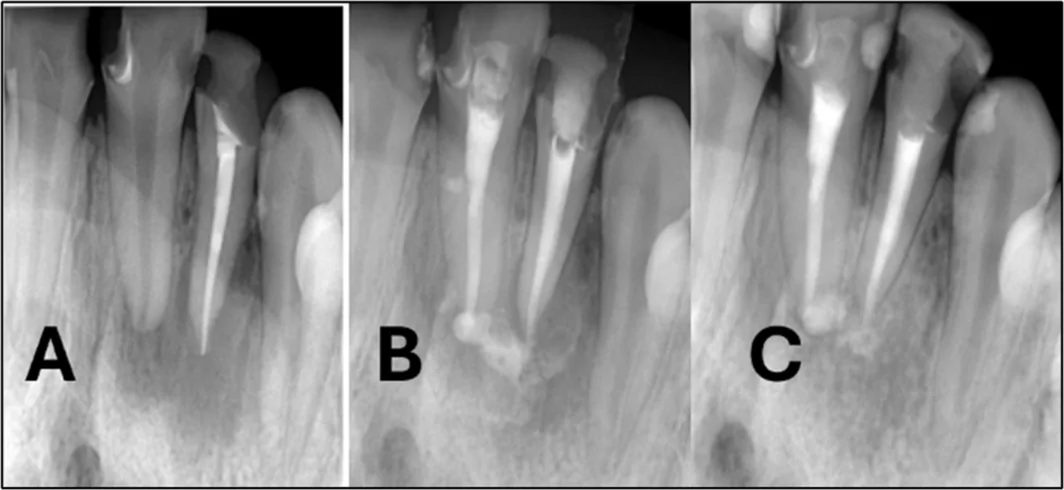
Case 2. (A) Preoperative periapical radiograph demonstrating a large periapical radiolucency involving teeth #23 and #24. (B) Immediate postoperative radiograph following placement of 5‐mm CC apical plugs, with extrusion of CC. (C) Two‐year follow‐up periapical radiograph showing that the previously observed periapical radiolucency was no longer evident and that the radiographic visibility of the extruded CC was reduced compared with the immediate postoperative image.
